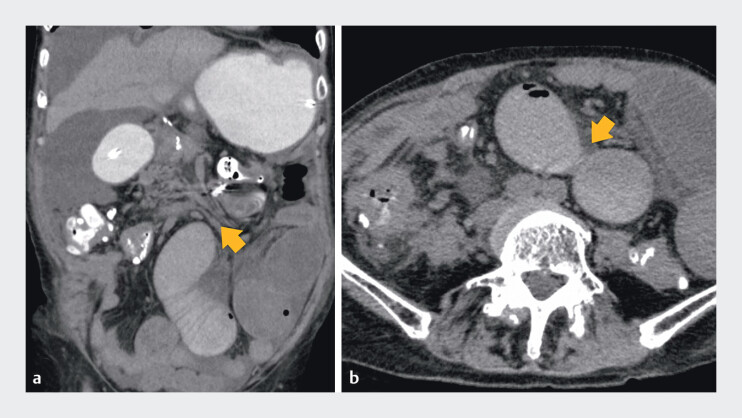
CT image showing a malignant stricture in the jejunum.
